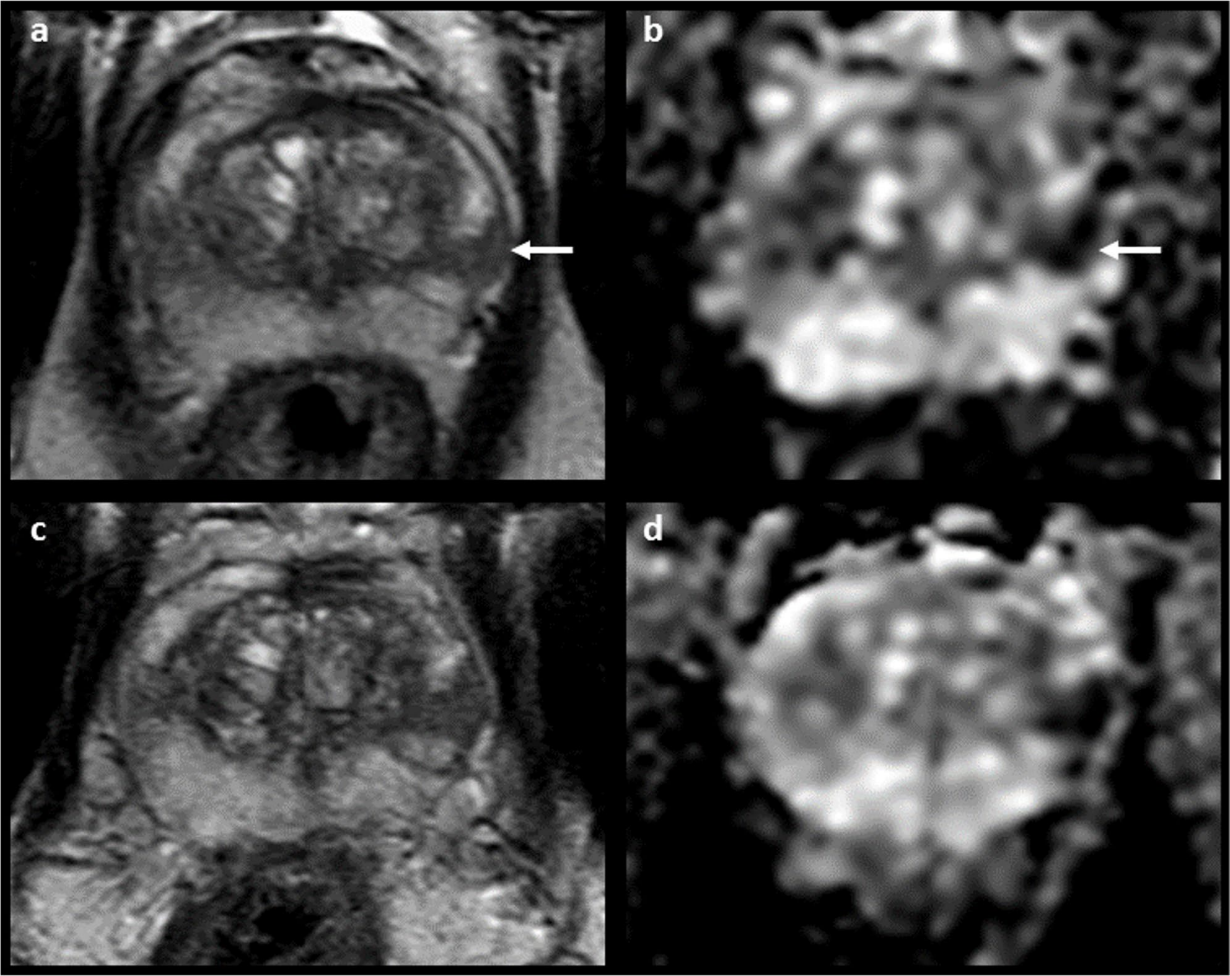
MRI positive, pathology negative: Targeting error. A 72-year-old, PSA 5.86 ng/mL. No prior biopsy. a, b: 10 × 8 mm PI-RADS 4 lesion in the left lateral mid-PZ (white arrows), showing low T2 signal (a) and focal restricted diffusion (b). Initial biopsy showed no malignancy but with 13/21 cores classified as inadequate, including 2/2 target cores. c, d Repeat MRI at 3 months confirms persistent high probability target, with subsequent repeat biopsy showing 2/2 target cores Gleason 3 + 4 = 7 (pattern 4 = 35%)
